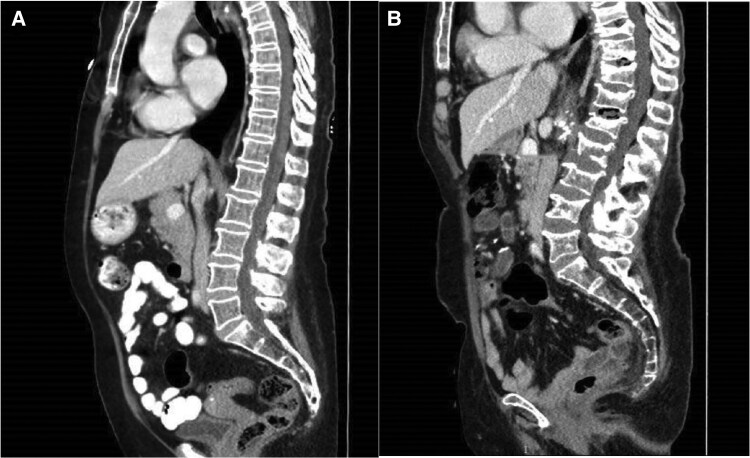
Computed tomography scans of the spine in a 70-year-old woman carried out several months apart demonstrating the occurrence of 9 vertebral fractures following cessation of denosumab after 5 doses. From Tripto-Shkolnik et al, Calcif Tissue Int, 103:44, 2018 (101), used with permission.
